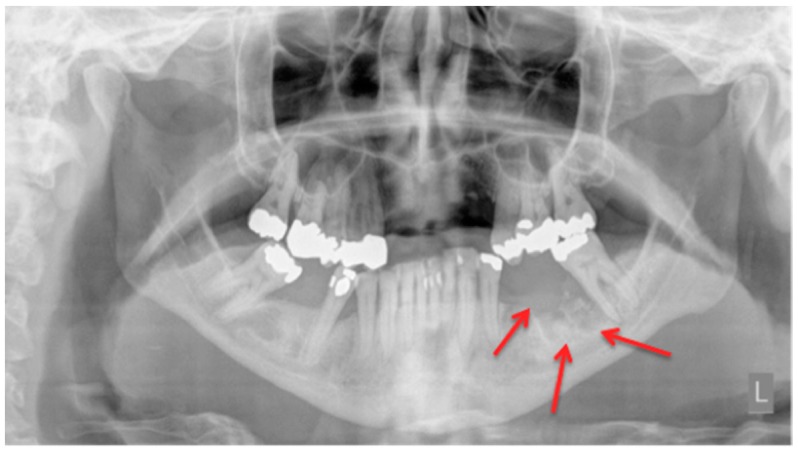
Panoramic radiograph: Patient: 48 years old, female, metastatic breast cancer, zoledronic acid. Red arrows point to the necrotic area. The corresponding magnetic resonance imaging (MRI) images are shown in Figure 6 and Figure 7. American Association of Oral and Maxillofacial Surgeons (AAMOS) staging: stage 2.
